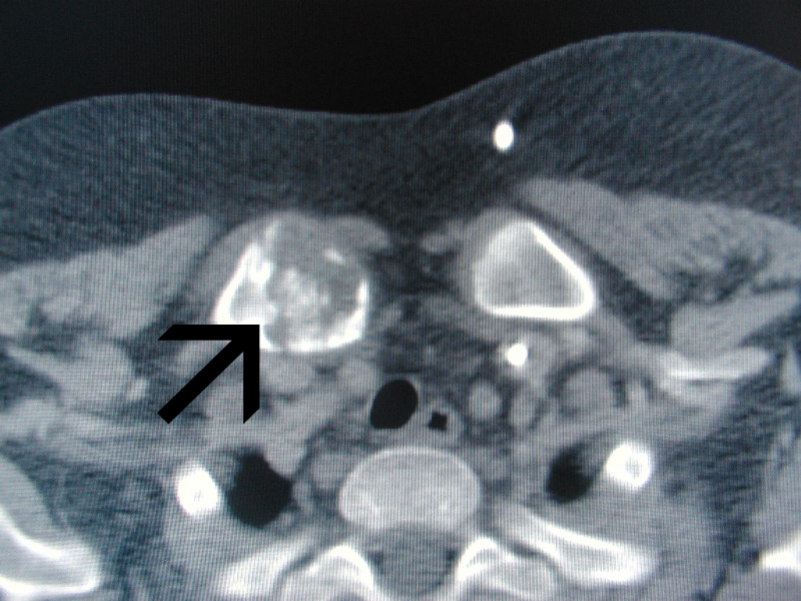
Computed tomography scan showing an osteolytic process with periosteal reaction of the right proximal clavicle.
